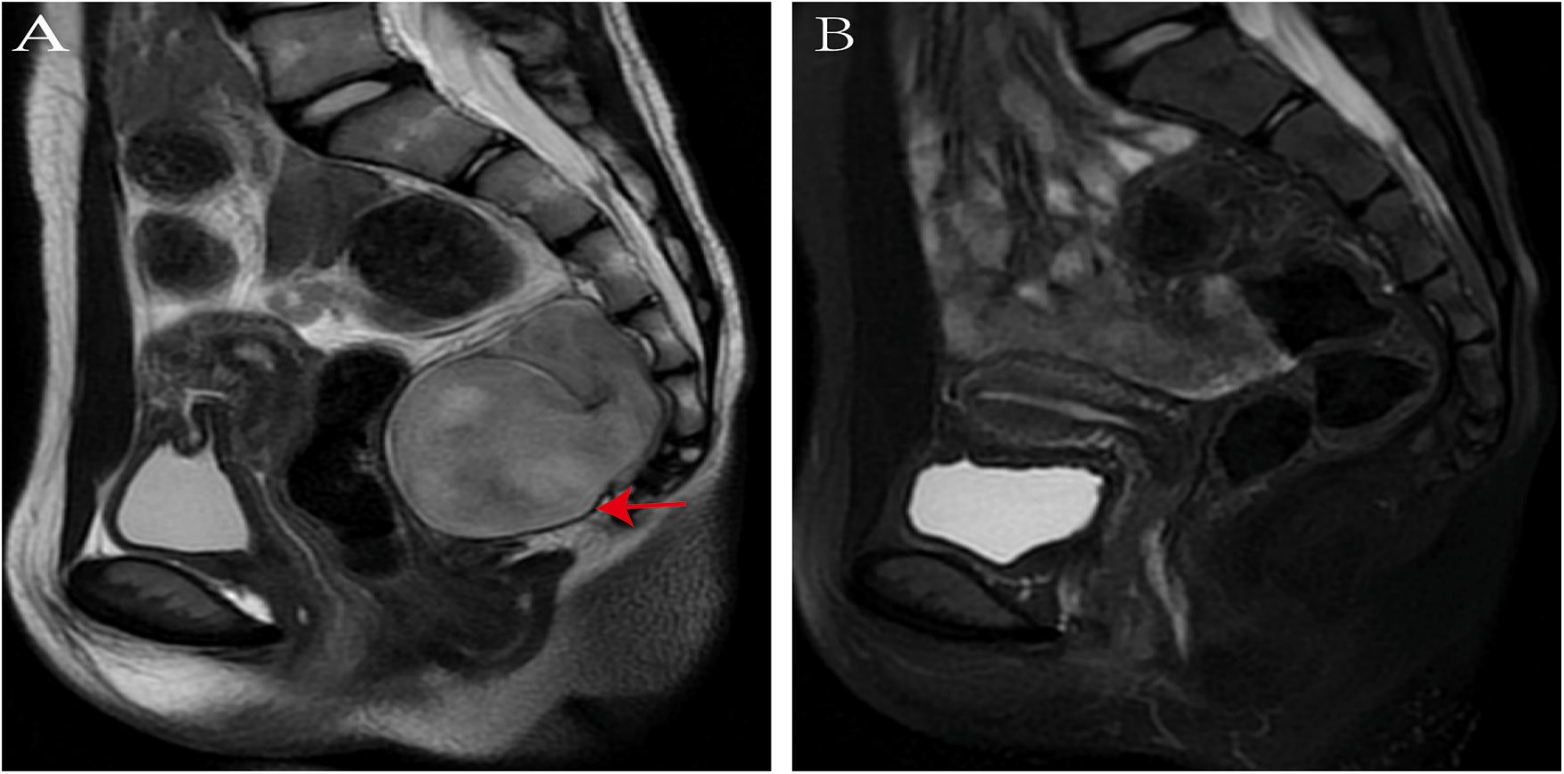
Preoperative and postoperative MRI findings (A) preoperative MRI; (B) postoperative MRI.
